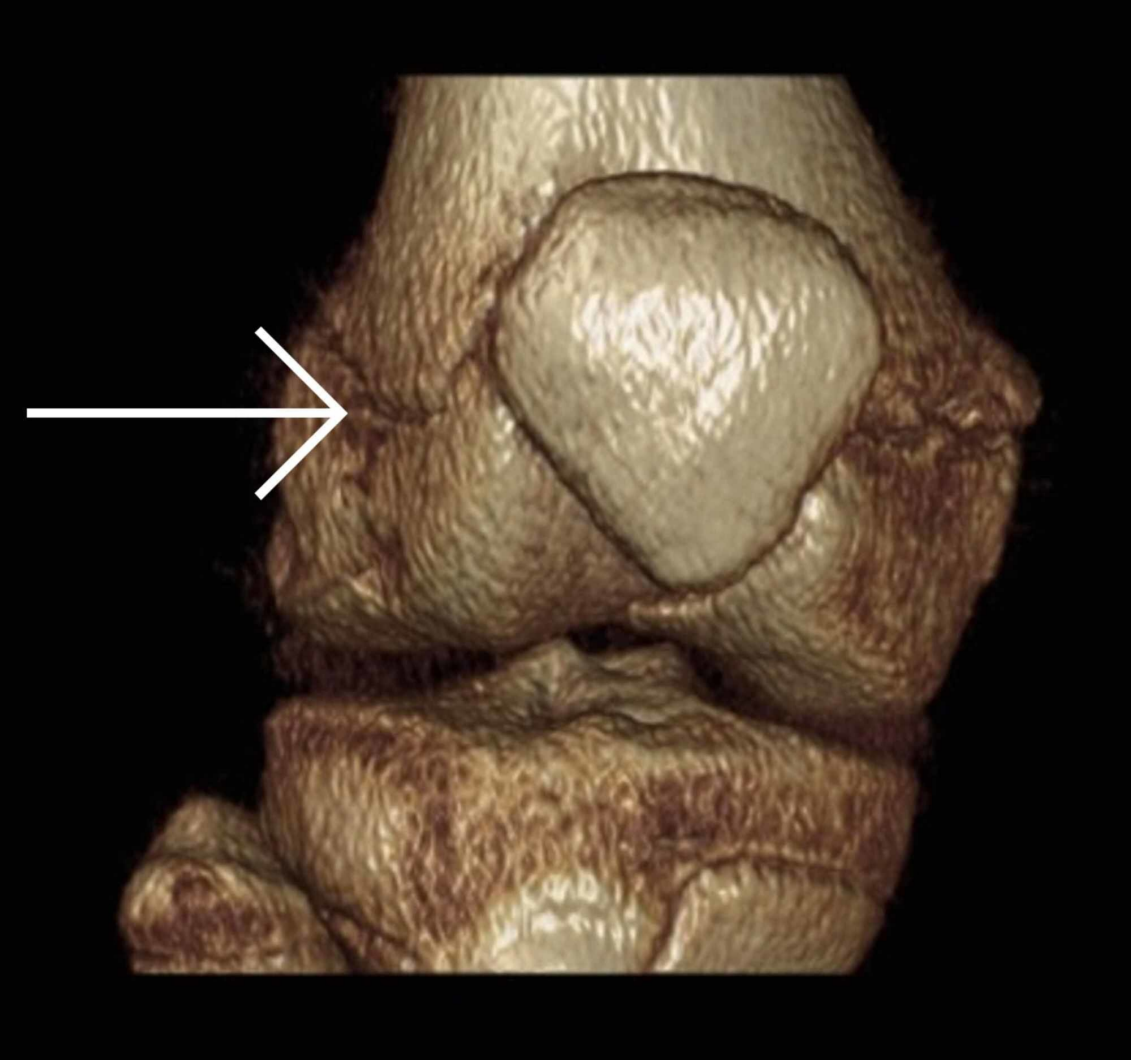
Coronal view of a computed tomography reconstruction post-injury
